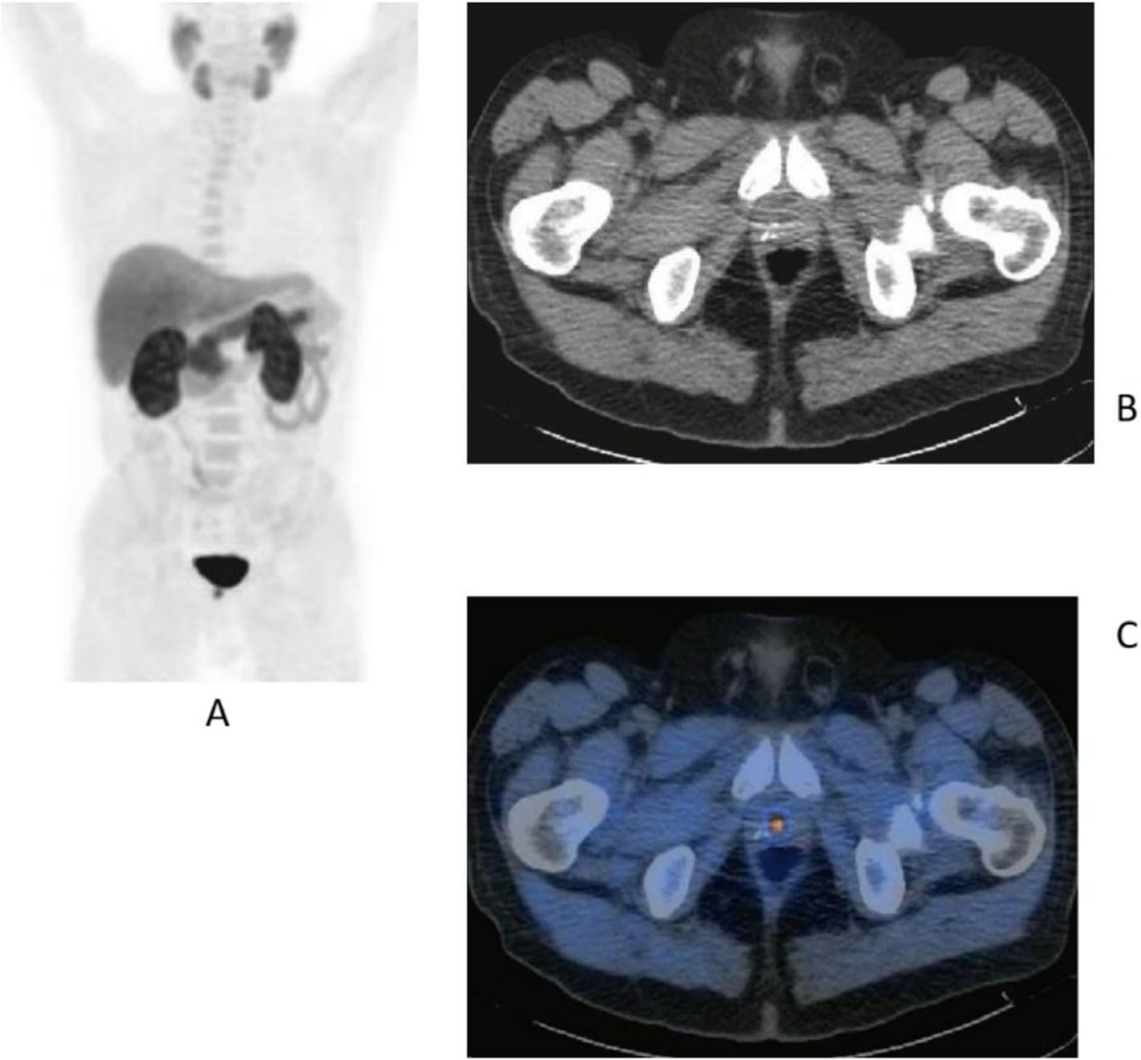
^18^F-fluorocholine, prostate cancer, biochemical recurrence (BCR). *Clinical history*: 72 y.o. man with prostate cancer, Gleason score 3 + 3, treated with radiation therapy. Patient underwent transuretral prostate resection prior to radiotherapy. BCR with a TTR 14 months and PSA 0.8 ng/ml PSAdt 13 months. *PET/CT findings*: focal increased uptake below the bladder seen on MIP (a), CT (b), and fused images (c)
